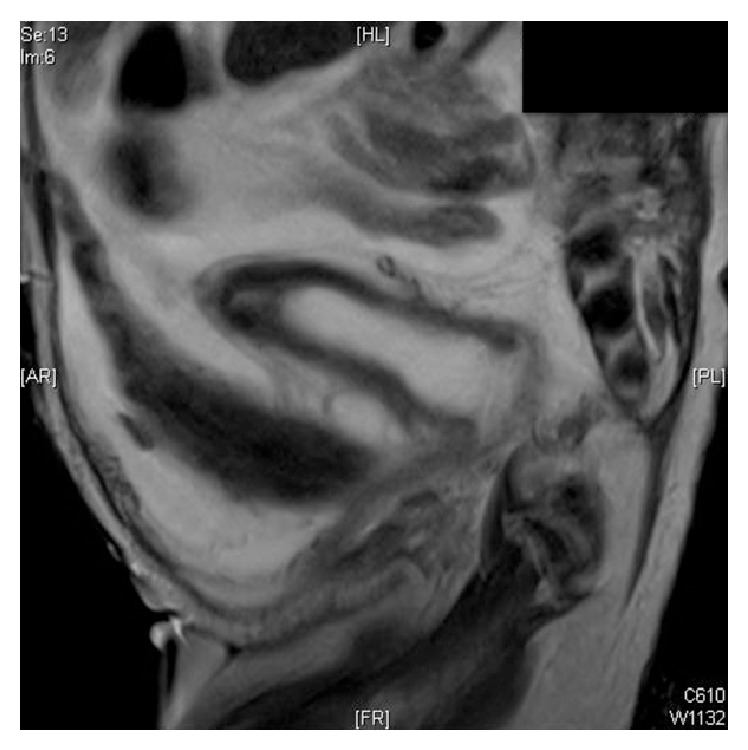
Pre-op MRI demonstrating an elongated bladder up to the level of urachus; a blind ending rectal pouch having no communication with the urogenital system; hydrometrocolpos; and absence of abdominal wall muscles.
